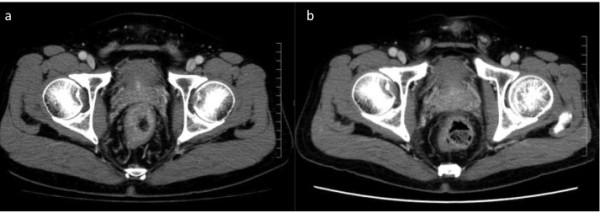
Computed tomography (CT) images. (a) CT imaging reveals rectal wall thickening and a regional lymph node metastasis in the mesorectum. (b) CT scan after chemotherapy demonstrates no rectal wall thickening and no mesorectal lymph node metastasis. CT, computed tomography.
